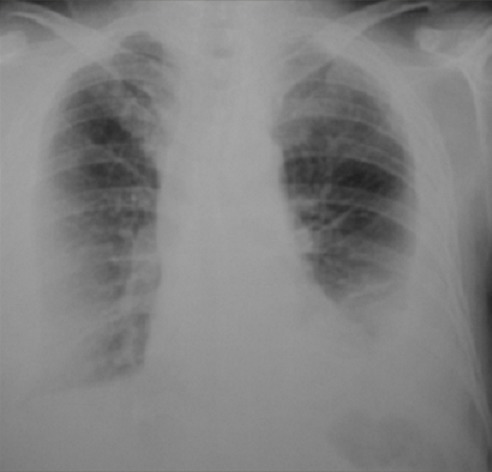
Radiographie du thorax: opacité bilatérale de type pleural en regression
